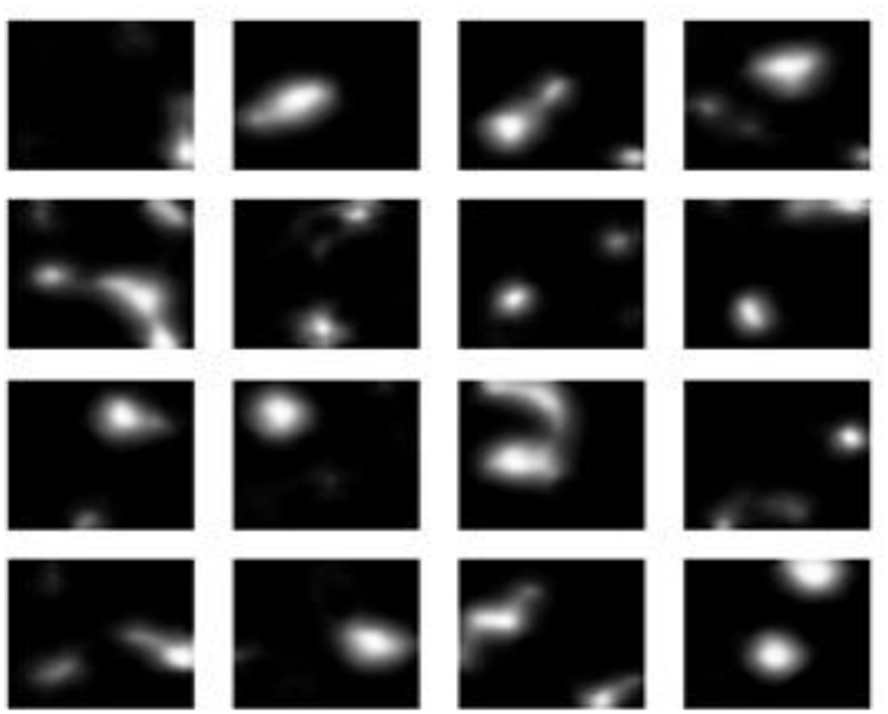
MRI generated by the cGAN model.
